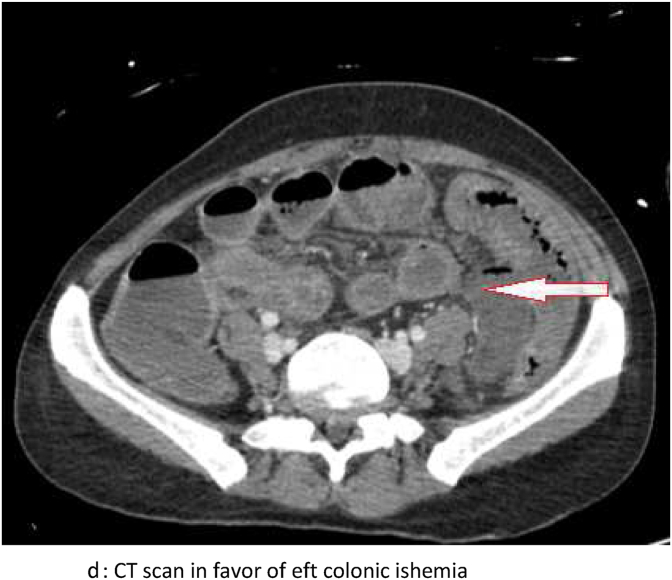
CT scan in favor of eft colonic ishemia.
